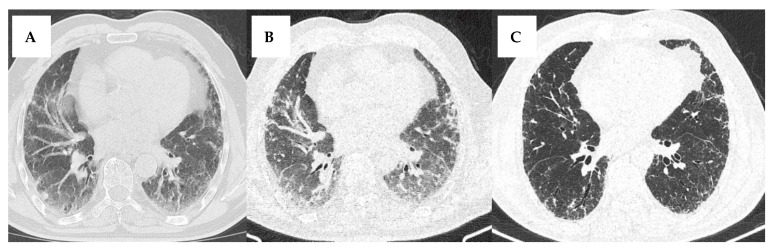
Impact of HRCT Acquisition Parameters on the Assessment of ILD: Comparison of Slice Thickness and Respiratory Phase. We present the case of a 71-year-old man who was evaluated in our clinic for a three-month history of dry cough and exertional dyspnea on moderate effort. He had previously undergone two imaging assessments in different centers, performed eight days apart. The first scan (A) was acquired in expiration, with a slice thickness of 2 mm. The second scan had a slice thickness of 0.6 mm and included images captured in both expiration (B) and inspiration (C). Findings include diffuse interstitial lung abnormalities with reticulation, ground-glass opacities, and traction bronchiectasis. When comparing the first two images (both acquired in expiration), the second image appears to have more defined lung structures. The 2 mm scan has a slightly more blurred appearance, reducing the visibility of small airway or parenchymal abnormalities. Furthermore, on the third image (acquired in inspiration), the lungs are more inflated, allowing better differentiation of true ground-glass opacities versus normal lung attenuation. In addition, airway structures appear less crowded, making it easier to assess traction bronchiectasis and the reticular pattern.
